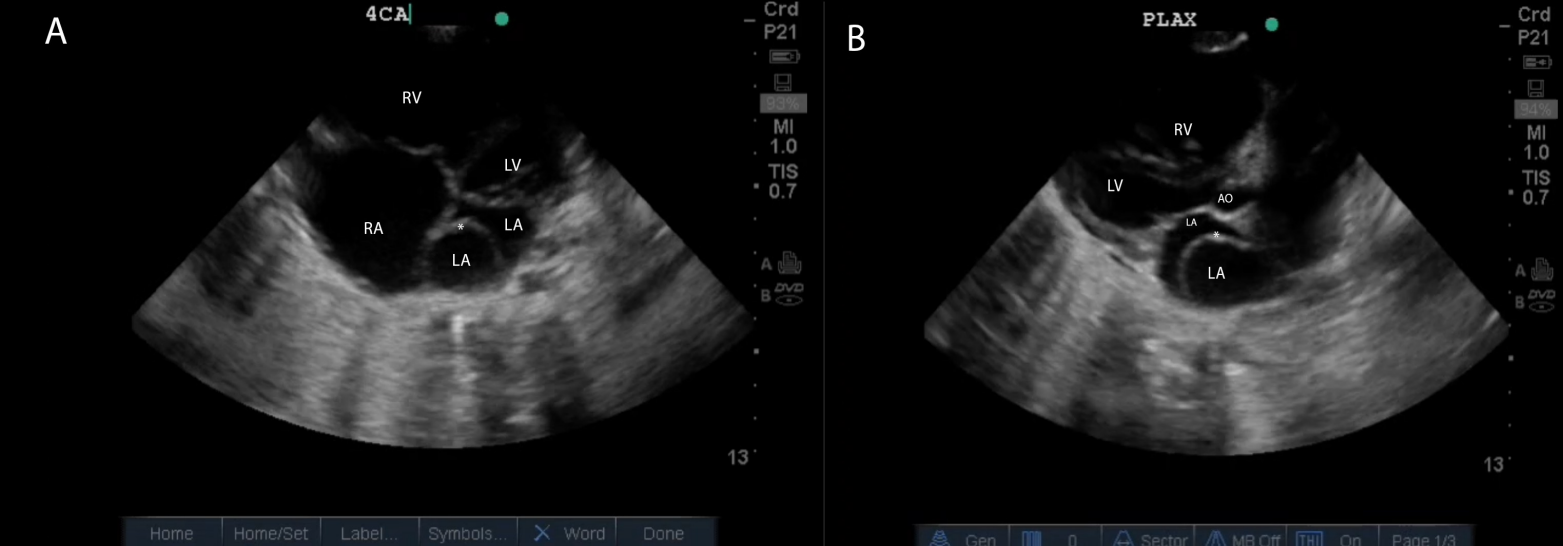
Cardiac point of care ultrasound (POCUS) showing Cor triatriatum. A. Apical four-chamber views (4CA) revealing a membrane. (White star) subdividing the left atrium into two chambers. B. Parasternal long axis view (PLAX), LA: left atrium; LV: left ventricle; RV: right ventricle; RA: right atrium; AO: Aortic root.
